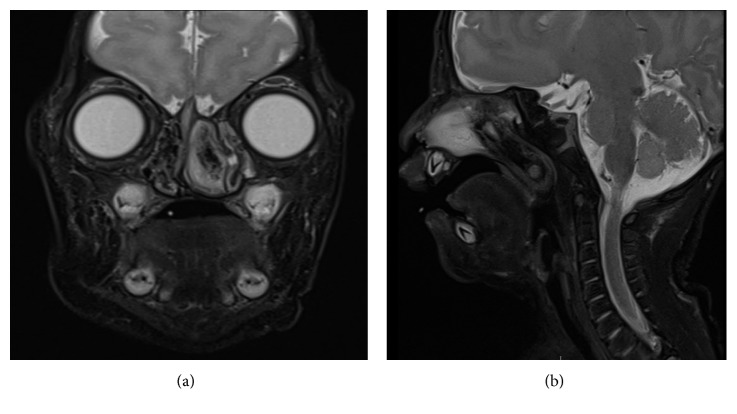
MRI T2-weighted axial (a) and sagittal (b) images depicting a left nasal mass arising from the roof of the nasal cavity and extending to the posterior choana, with no intracranial extension seen.
